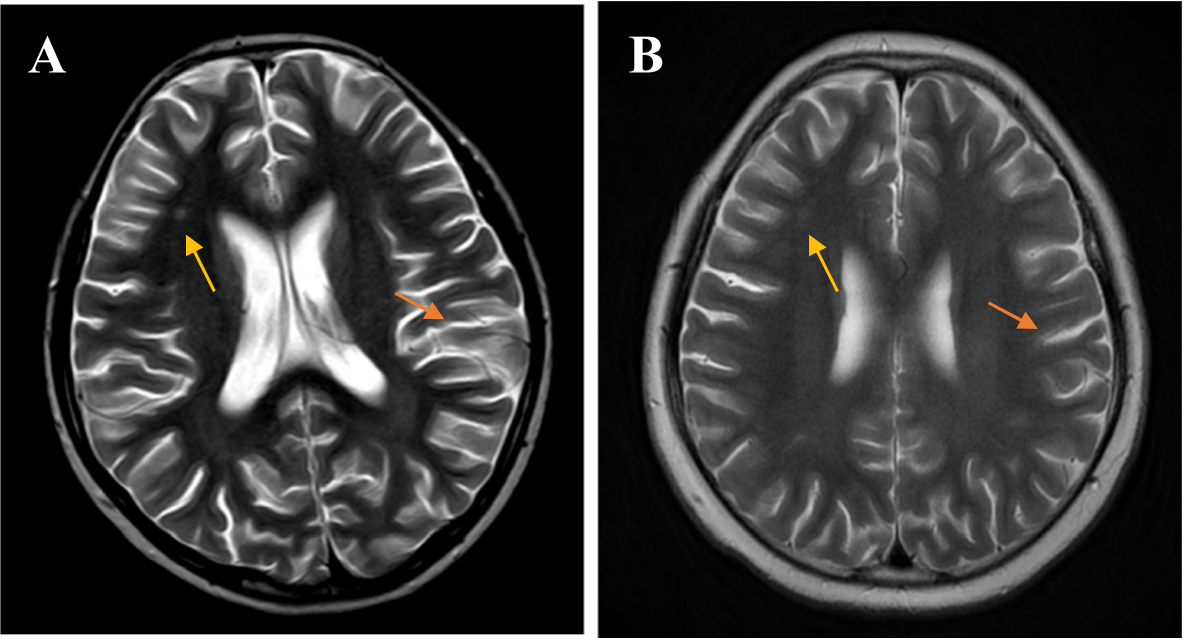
Comparison of T2-weighted head magnetic resonance imaging (MRI) before (A) and after (B) treatment of CYC. A A high-intensity dot zone in the right frontal white matter and high-intensity flake zone in the left temporal lobe can be seen. B There is no significant change of the high-intensity dot zone in the right frontal white matter and improvement of the high-intensity flake zone in left temporal lobe compared with before
